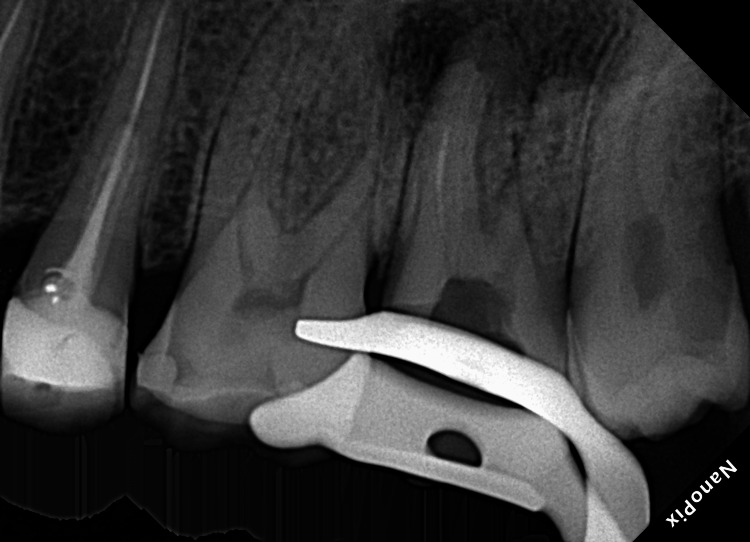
Intermediate radiograph after removed extruded gutta percha and separated file.
